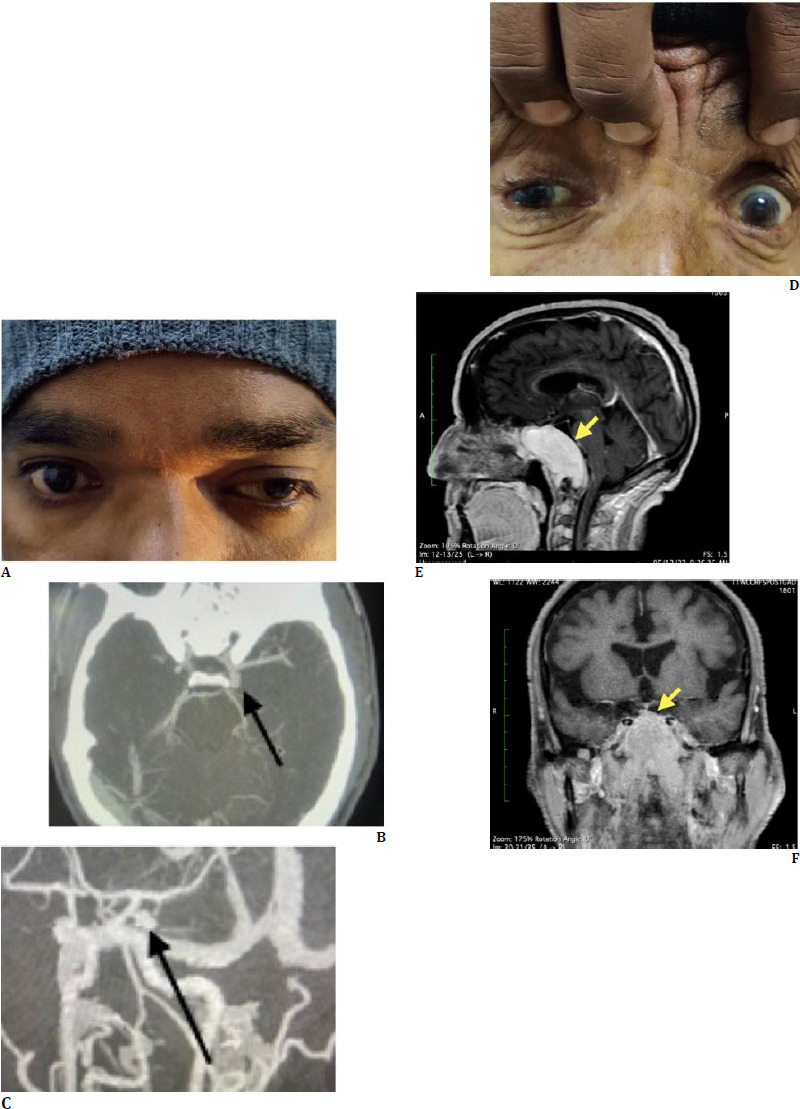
A. Left eye of a young male shows mild ptosis with down and out appearance in primary gaze; B and C CT Angiography reveals bilobed aneurysm (black arrow) arising from the terminal left ICA that is directed posteriorly; D. Right eye of an elderly male, presenting with complete ptosis (image not shown), shows down and out appearance in primary gaze; E. Post contrast T1 sagittal section shows intense homogenous enhancement of a lobulated mass epicentered in the clivus; F. Coronal T1 image shows intense homogenous enhancement that is involving bilateral cavernous sinuses. The lesion is also encasing the bilateral internal carotid arteries (ICAs). A few foci of blooming are also visible (images not shown) in the lesion
